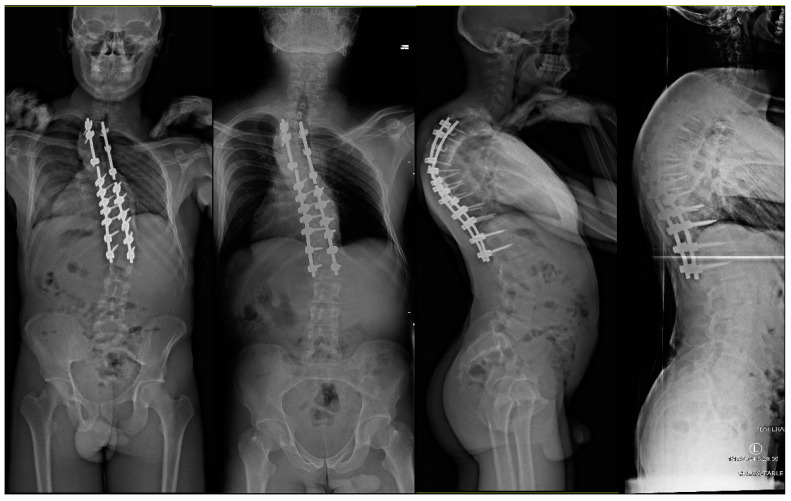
25-year-old male who previously underwent a posterior spinal instrumented fusion (PSIF) for T2-L1 at the age of 17 years presented with progressive back pain in the setting of bilateral rod fractures from T6 to T8. Preoperative anterio-posterior (AP) standing full-body radiographs demonstrated an 82° proximal thoracic and 65° main thoracic curve. Supine images showed the proximal and main thoracic curves decreasing to 77° and 48°, respectively. Preoperative lateral standing full-body radiographs demonstrated 88° of thoracic hyperkyphosis, 78° of hyperlordosis, and a small pelvic incidence of 48°.
